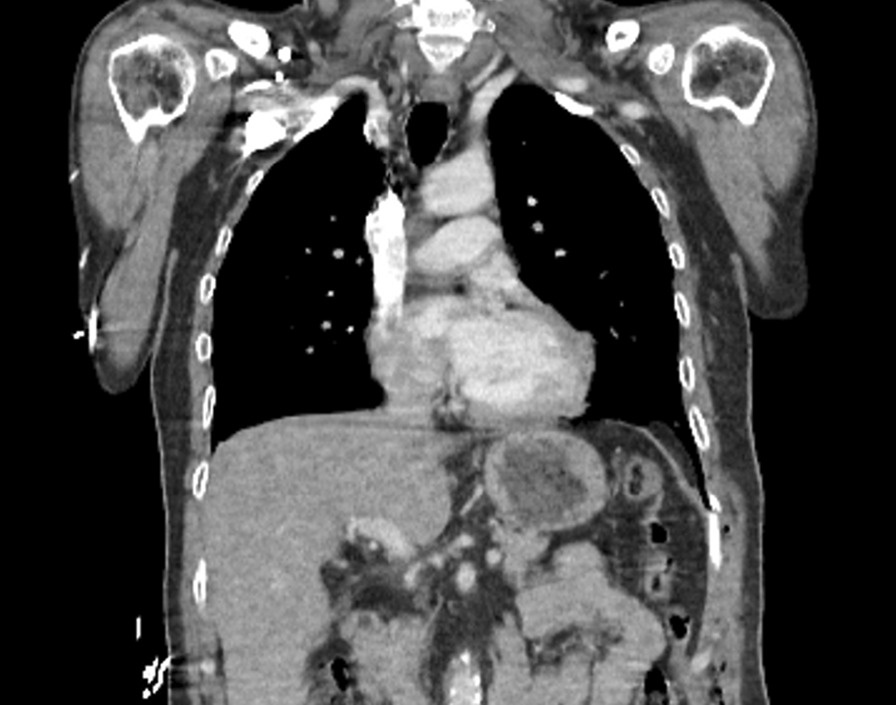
Coronal view of a thoracoabdominal computed tomography scan, after a stab wound 3 months before the diaphragmatic hernia. There is no significant diaphragmatic defect and apparent diaphragmatic hernia
